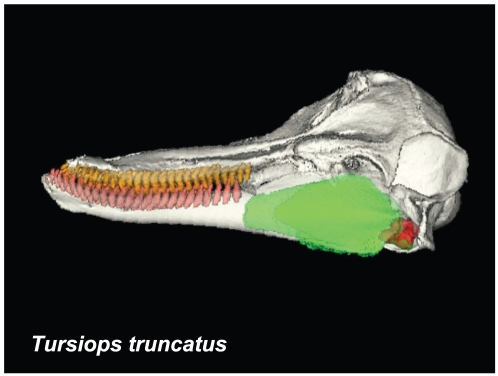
Left lateral view of the Atlantic Bottlenose Dolphin (*Tursiops truncatus*) from CT reconstructions. Skull = ivory, maxillary teeth = orange, mandibles = ivory, mandibular teeth = salmon, left mandibular fat body = green, left TPC = red.
